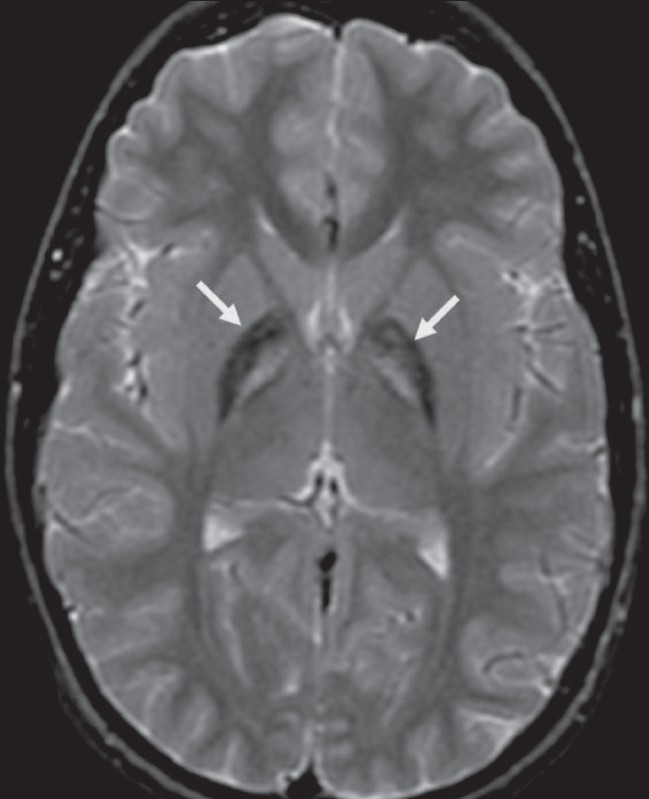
The eye-of-the-tiger sign. Axial T2W MRI image of the brain shows hypointensity of the globus palladi (arrows). There is relative hyperintensity of the central part, giving the globus palladi the appearance of the eyes of a tiger. This appearance is seen in Hallervorden-Spatz syndrome
